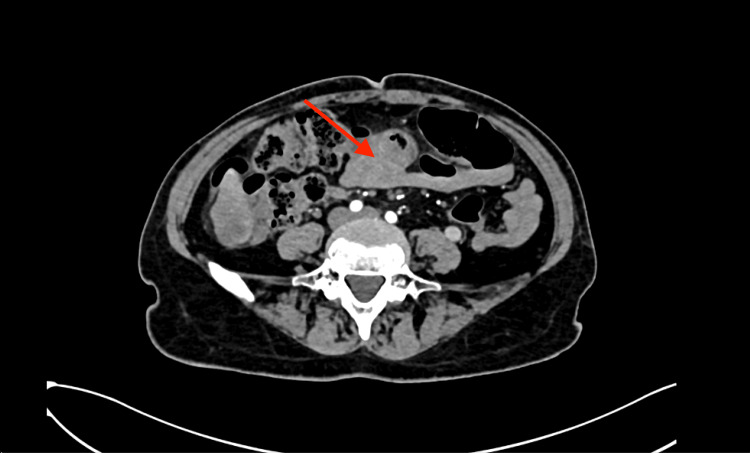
CT of the abdomen showing transversal colon mass (red arrows)
